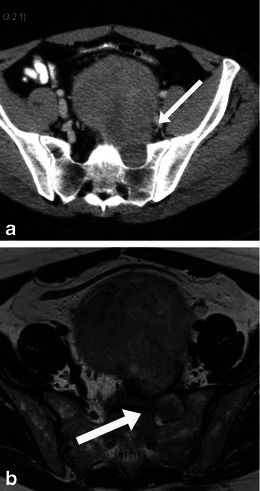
Imaging of cellular schwannoma. Axial contrast-enhanced CT scan (a) and axial T2-weighted MRI scan (b) show a heterogeneously enhancing soft-tissue mass (*arrows*) in a 26-year-old woman. The left S2 neural foramina is widened with bony destruction
